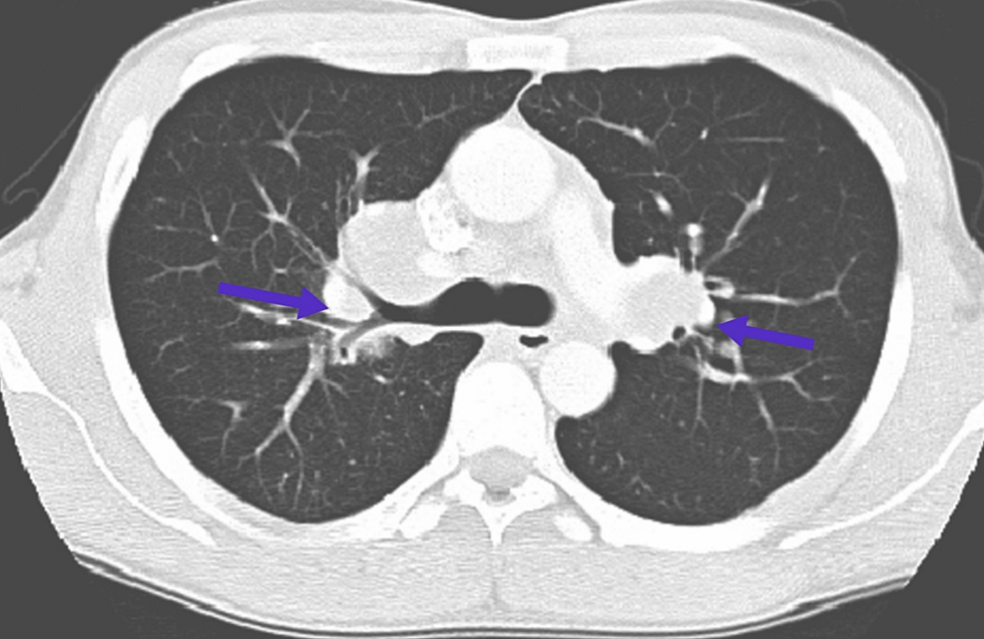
CT of the chest. Blue arrows show bilateral hilar lymphadenopathy.
